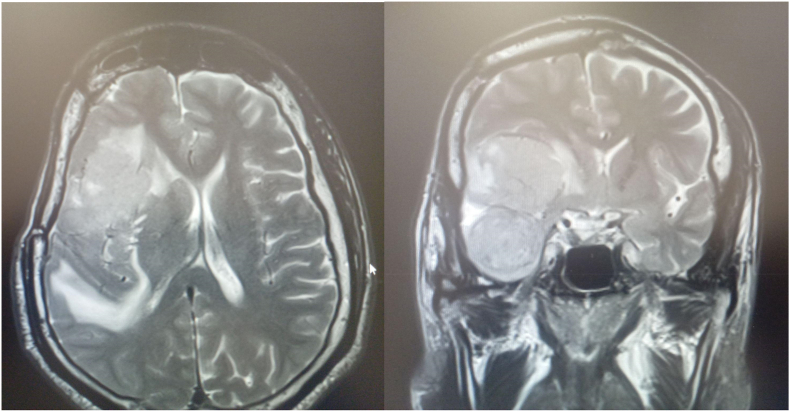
MRI brain T2 sequence showing a right sylvian fissure meningioma with surrounding perifocal edema.
